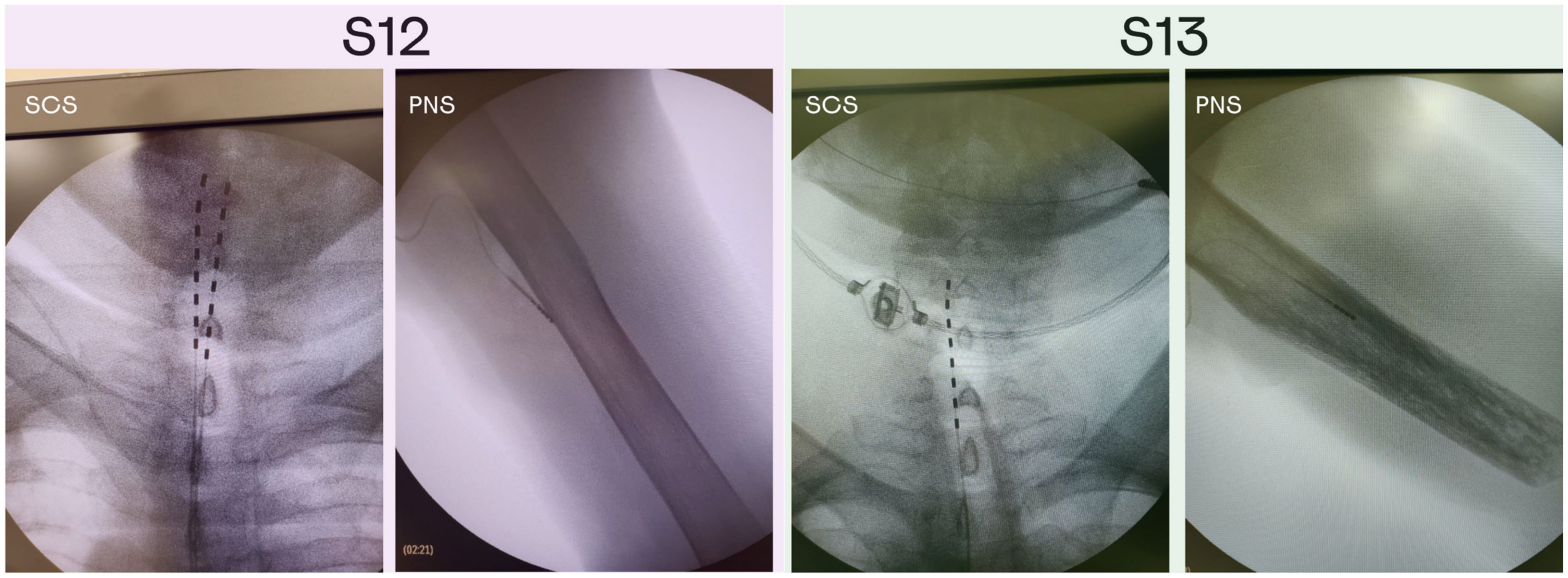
X-ray images of the PNS and SCS electrodes position in patients S12 and S13. Patient S12 was implanted with two electrodes in the area of intumescentia cervicalis of the spinal cord and one electrode in the medial nerve of the left hand. Patient S13 was implanted with two electrodes in the area of intumescentia cervicalis of the spinal cord and one electrode in the medial nerve of the left hand.
